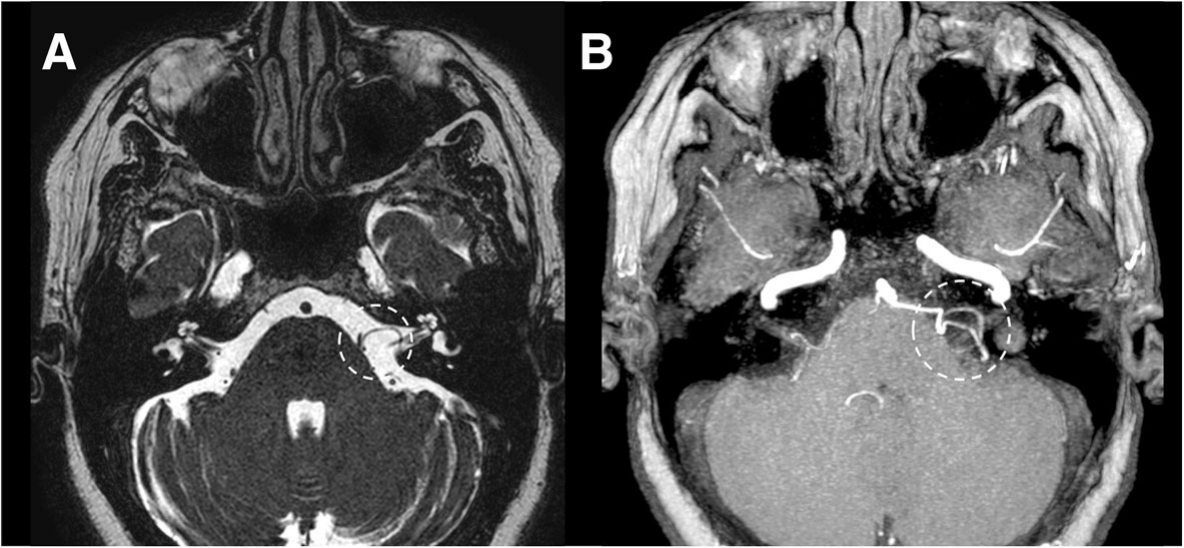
Neurovascular compression of facial nerve. Patient with left facial nerve palsy. MRI axial steady-state free procession (a) and T1-weighted post-gadolinium, MIP reconstruction (b) images show a neurovascular compression of the left facial nerve (dotted circles)
